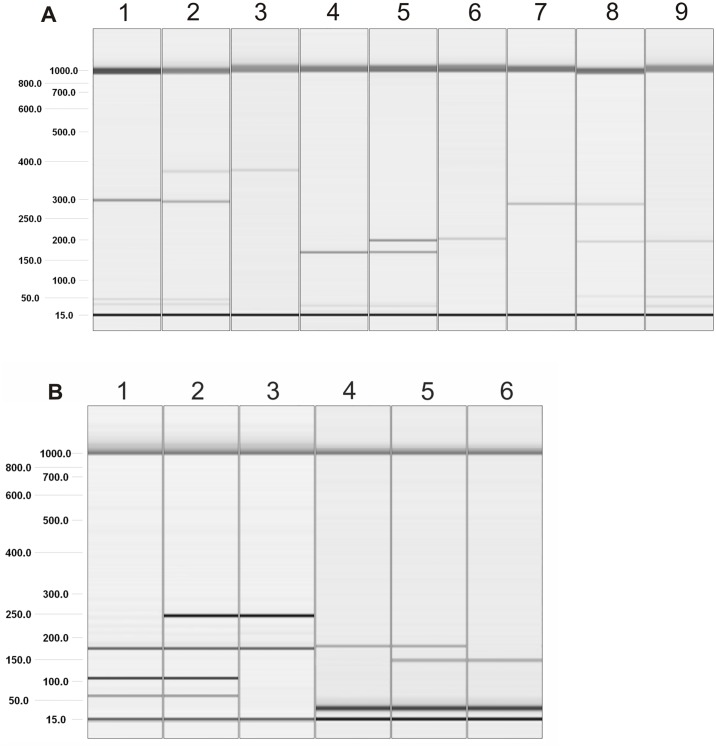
Visualization of PCR-RFLP products for *TLR3* SNP (A) and *TLR7* SNP (B) genotyping. Gel image: A) rs3775290: 1. wild type CC, 2. heterozygous CT, 3. homozygous recessive TT; rs3775291: 4. wild type CC, 5. heterozygous CT, 6. homozygous recessive TT; rs3775296: 7. wild type CC, 8. heterozygous CA, 9. homozygous recessive AA. B) rs179008: 1. wild type AA, 2. heterozygous AT, 3. homozygous recessive TT; rs5741880: 4. wild type GG, 5. heterozygous GT, 6. homozygous recessive TT. Alignment markers (15 bp, 1 kbp).
